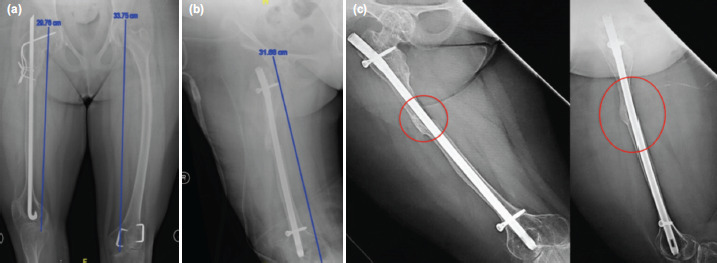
(a) Pre-operative radiograph with estimated 4cm shortening and malrotation, evidence by the overlapping distal femoral condyles in anteroposterior view. (b) Post-operative radiographs with bone gap of 2cm filled with iliac bone cortical graft. (c) Post-operative AP and Lateral radiograph showing well incorporated bone graft.
